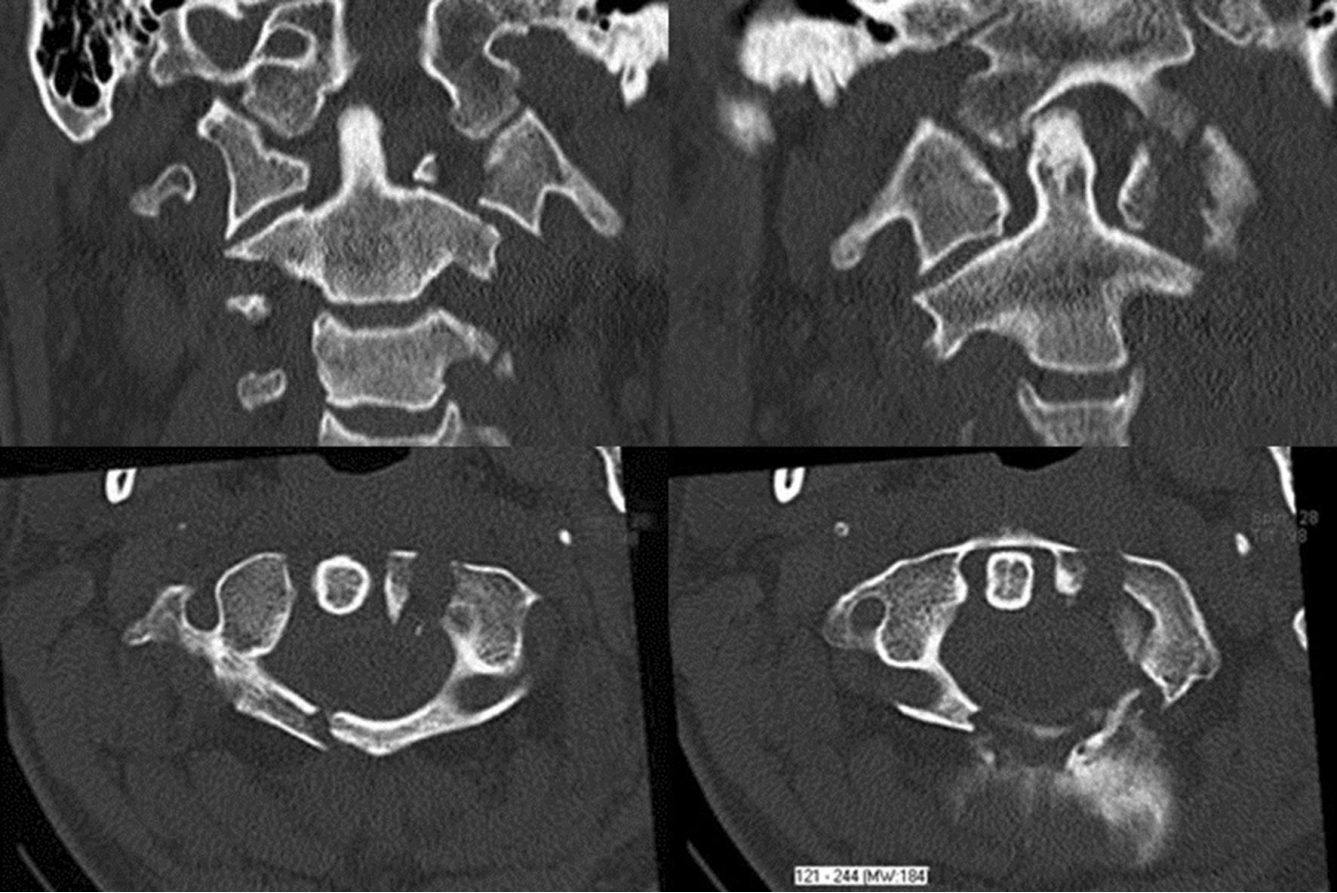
Coronal (*top*) and transversal (*bottom*) CT images of patient 3: Jefferson type IV (Landells and Van Peteghem type III) and posterior arch fracture with dislocation of the left lateral mass
